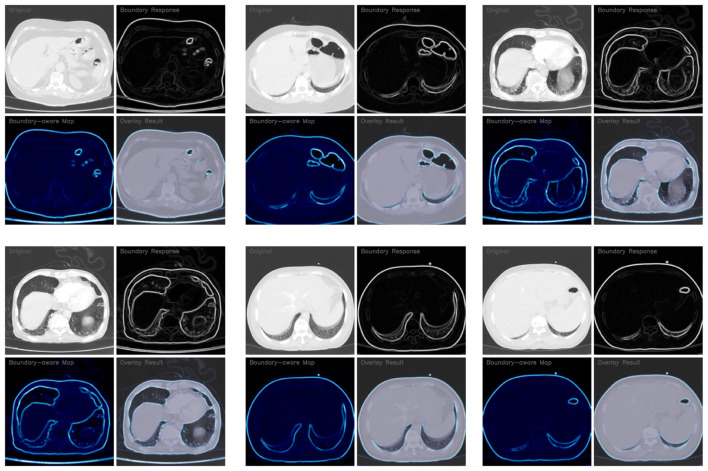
Visualization results of vascular boundary-aware representations on representative CT images, including the original image, boundary response, boundary-aware map, and overlay result, showing how the model captures structurally relevant boundary information in pulmonary embolism CT analysis.
